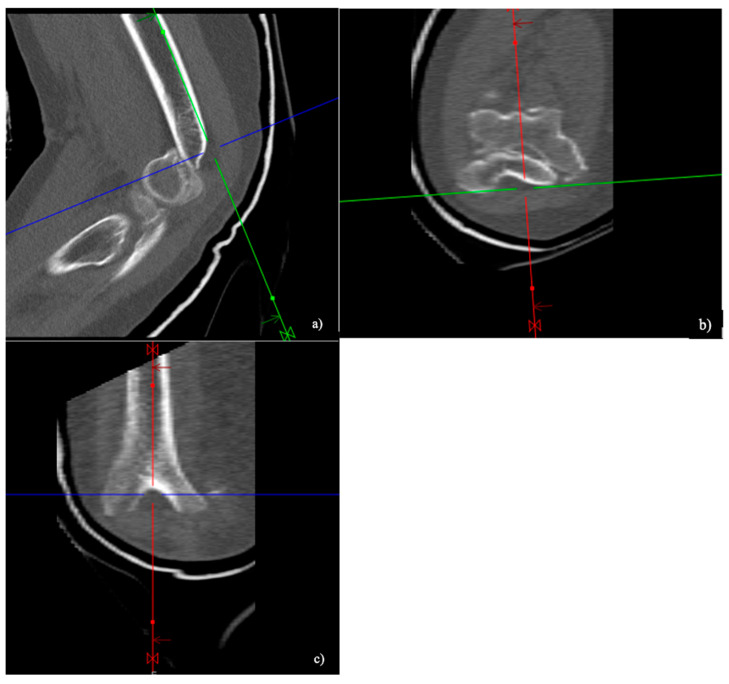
Computer tomographic (CT) images of a ‘low-plane’ distal humerus fracture in the sagittal (a), axial (b), and coronal (c) reconstruction.
